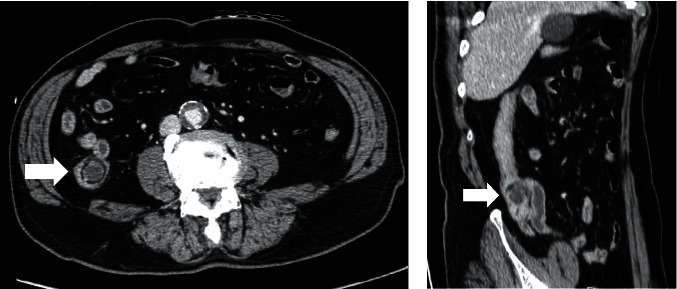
Contrast-enhanced axial (a) and coronal oblique (b) computed tomography of the abdomen shows a “bowel-in-bowel” appearance (white arrows) in the right lower quadrant. This appearance was consistent with ileocecal intussusception, and there was no evidence of proximal obstruction.
